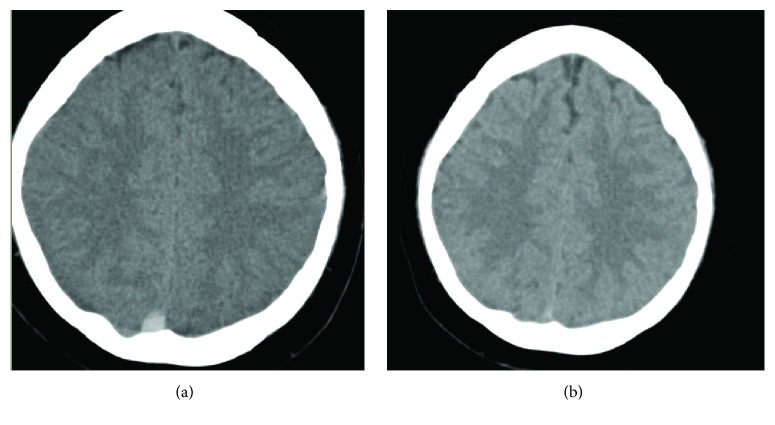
Unenhanced CT scan of the head (a) showing acute hyperdense thrombus close to the torcula and (b) complete resolution 4 months later following therapeutic anticoagulation.
